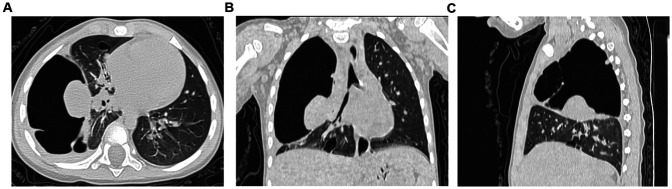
Chest CT plain scan performed on the day of admission. (A) Axial chest CT plain lung window; (B) Coronal chest CT plain lung window; (C) Sagittal chest CT plain lung window.
